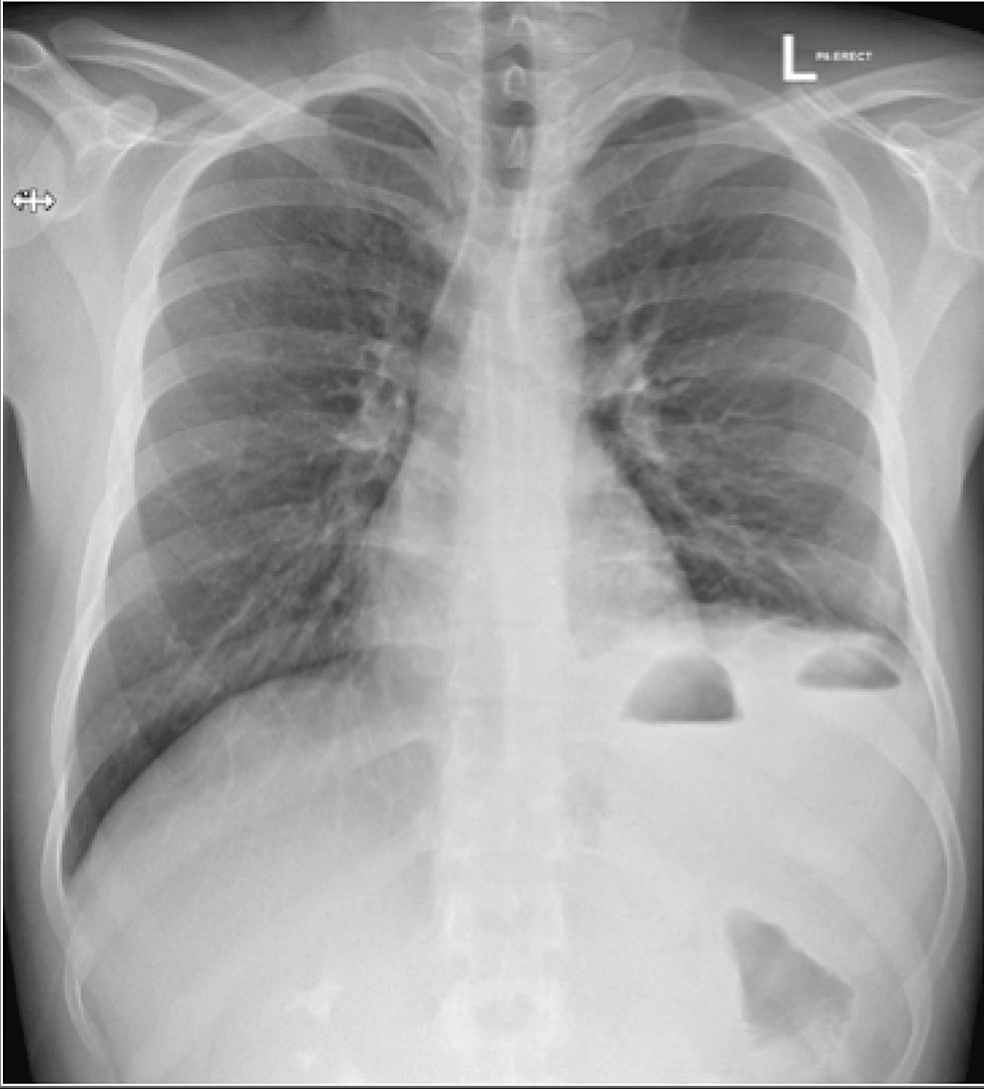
Chest X-ray showing an elevated left dome of the diaphragm with small air-fluid levels
